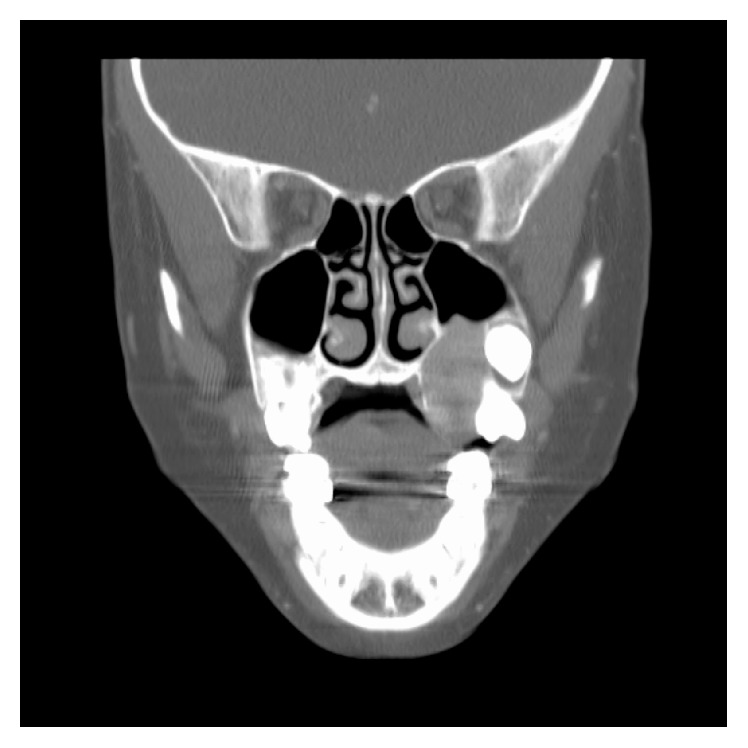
CT scan of the maxillofacial region in coronal view showing a destructive lesion of the left hard palate growing into the maxillary sinus.
